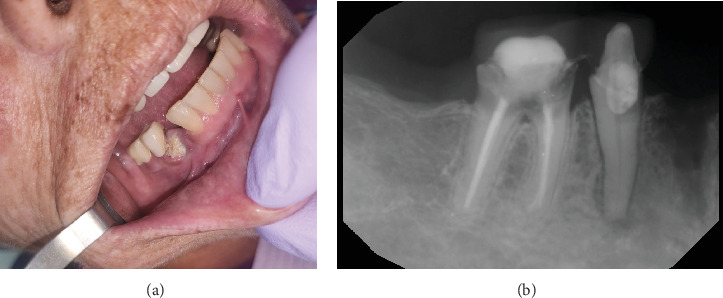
A 57-year-old female with medication-related osteonecrosis of the jaw. (a) The exposed necrotic bone of the right mandibular alveolar ridge measuring 10 mm × 10 mm in size and (b) periapical radiograph showing a nonhealing extraction site of #28 area of a year duration, secondary decay and periapical radiolucency of Tooth #29, and secondary decay with furcation involvement of an endodontically treated Tooth #30.
